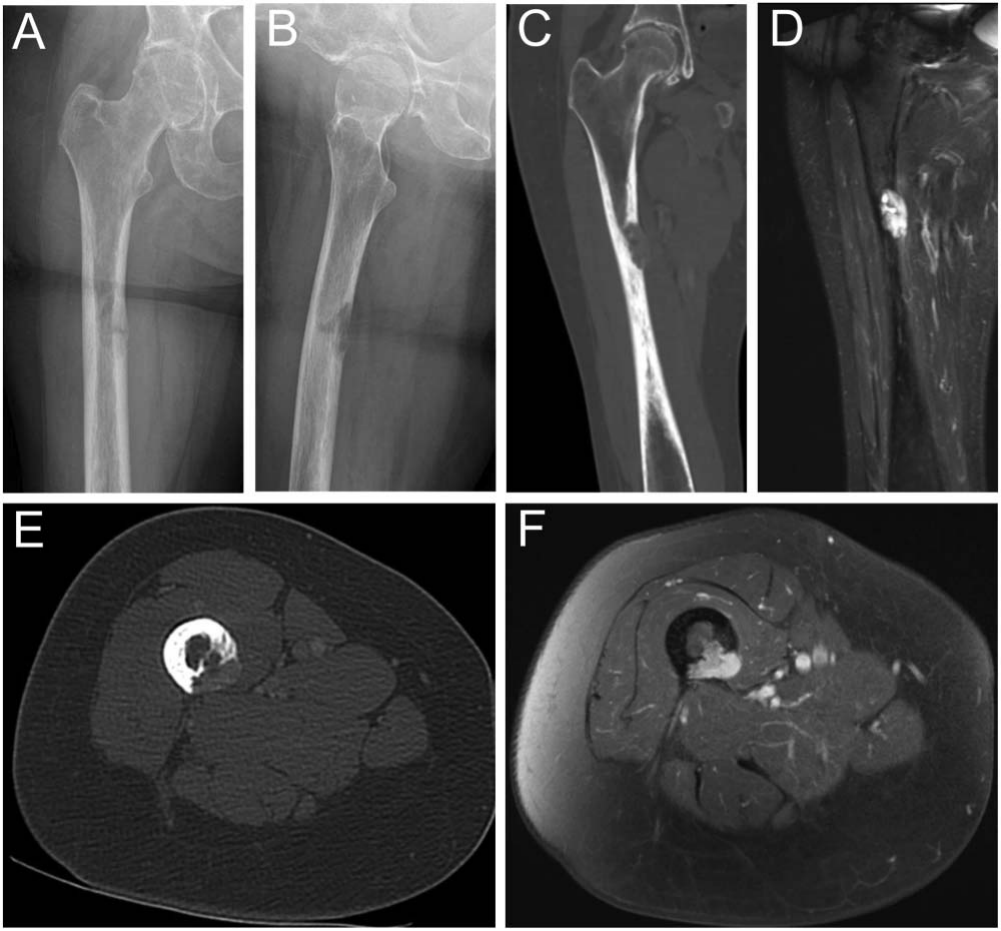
Radiological images using X-ray, CT, and MRI. (A and B) X-rays showing an osteolytic lesion with cortical erosion, accompanied by a pathological fracture. (C and E) CT scans demonstrating the tumor lesion with cortical bone destruction. MRI scans showing characteristic T2 hyperintensity and contrast enhancement (D), along with extraosseous soft tissue tumor formation (F).
